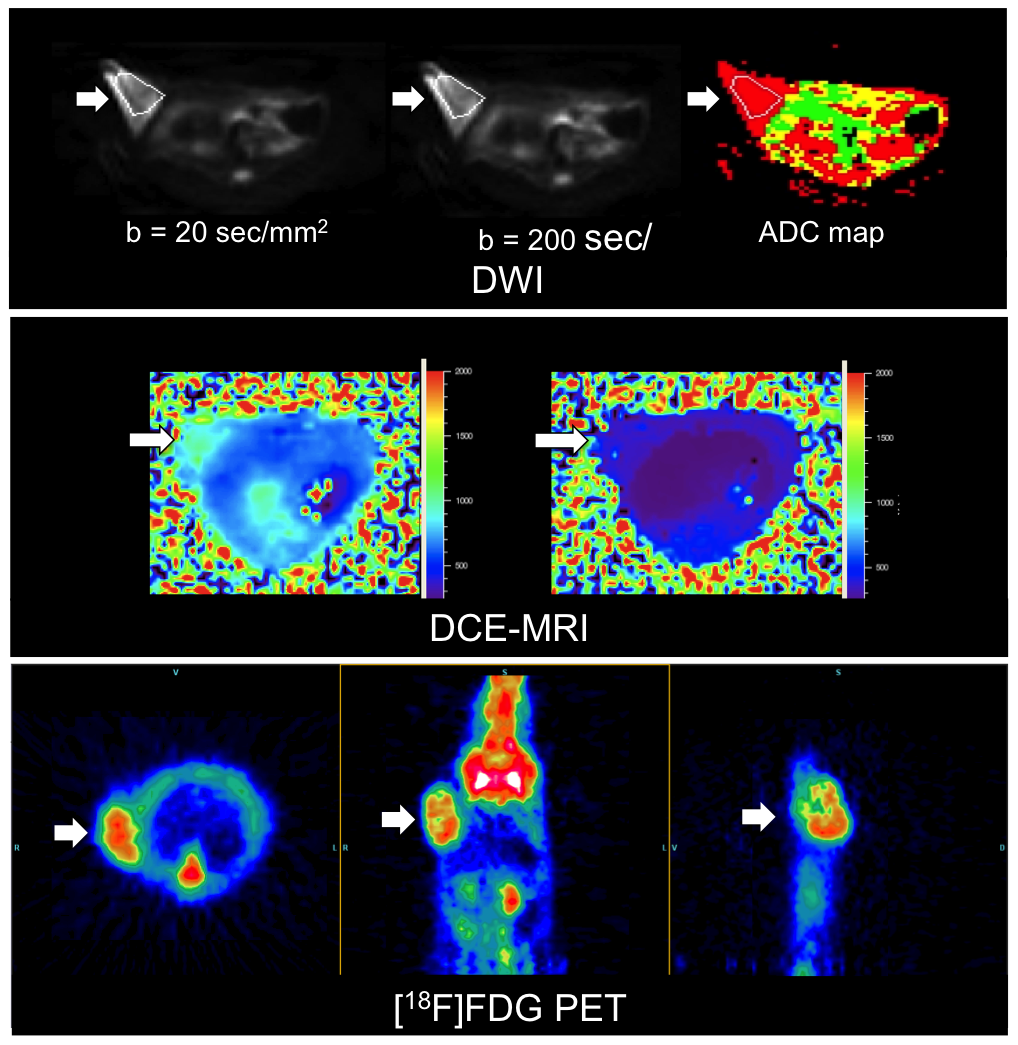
Representative DWI (transverse slices; b = 20, b = 200, ADC map), T1 map (T1 values are shown in color scale (ms)) of DCE-MRI from a single time point (transverse slices; pre and post i.v. injection of Gadolinium) used for T1 quantification and FDG-PET (from left to right: transverse, coronal and sagittal reconstruction) images of the A673 rhabdomyosarcoma xenografts (arrows) subcutaneously implanted in the right lateral flank.
